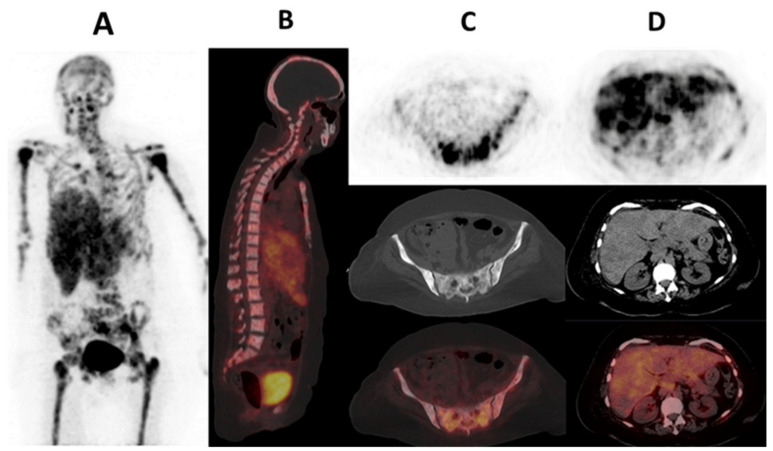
A 51-year-old woman known with metastatic invasive lobular breast cancer estrogen and progesterone receptor-positive, and HER2-negative and a Ki-67 of 45%. She had a bilateral modified radical mastectomy with disease progression despite chemotherapy. She had [^68^Ga]Ga-FAPi-04 PET/CT performed which showed diffuse skeletal metastases (A–C) in a pattern consistent with bone marrow distribution as well as liver metastases (D).
